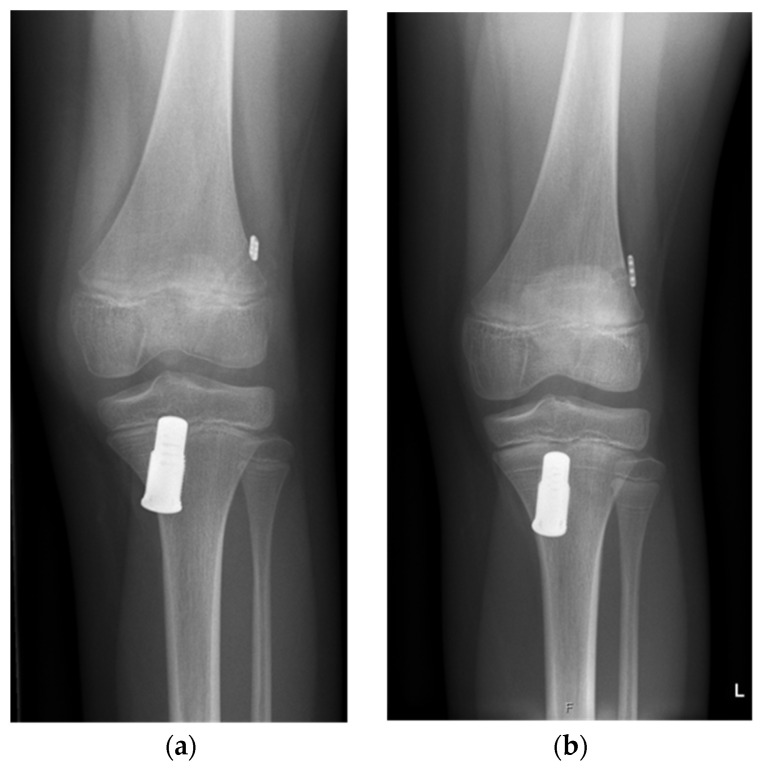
Anterior–posterior radiographs of a knee of an eleven-year-old girl who underwent dynamic intraligamentary stabilization repair. (a) Initial postoperative radiograph; (b) one year postoperative: Due to the growth of the patient (symmetric Harris growth line), the Ligamys screw is now located more distally. The physis of the proximal tibia is still open.
